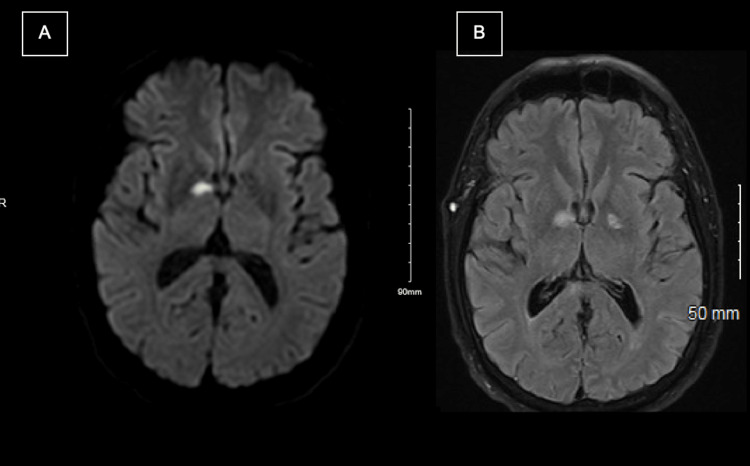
MRI Brain without contrast: A) DWI sequence with an evident focal restricted diffusion in the right basal ganglia; B) T2/FLAIR sequence showing a hyperintense lesion in the same region consistent with subacute right basal ganglia infarction. DWI: Diffusion-weighted imaging FLAIR: Fluid attenuated inversion recovery
